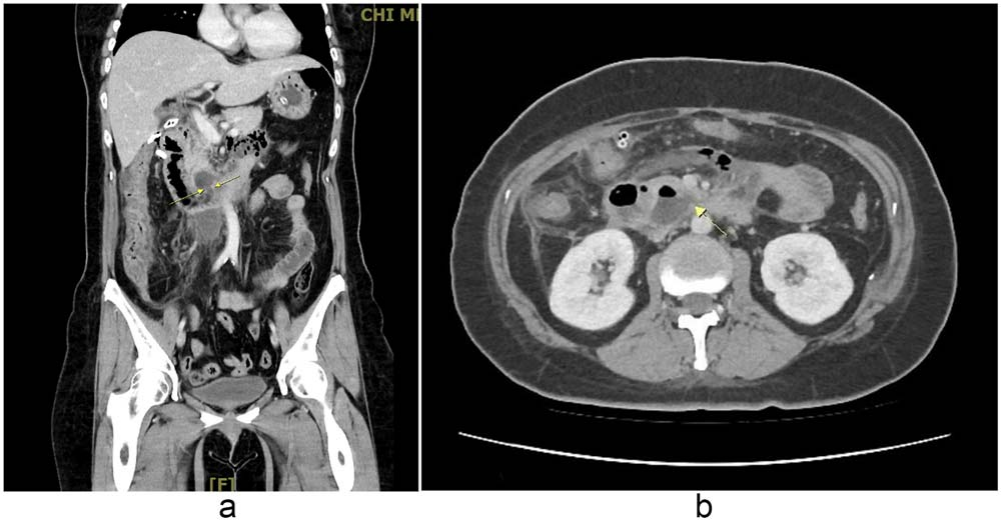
(a, b) Contrast-enhanced abdominal CT scan (coronal and axial views, 2 September 2025). Imaging demonstrates extraluminal air bubbles and a retroperitoneal abscess (arrow) adjacent to the third portion of the duodenum, consistent with duodenal perforation.
